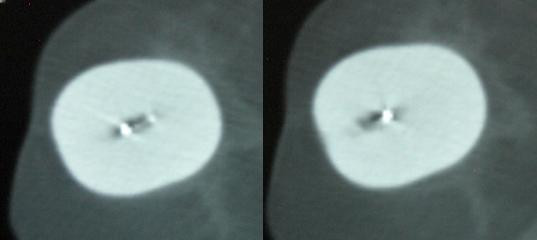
CT scan slices from case 2 showing the asymmetry of the center channel.
